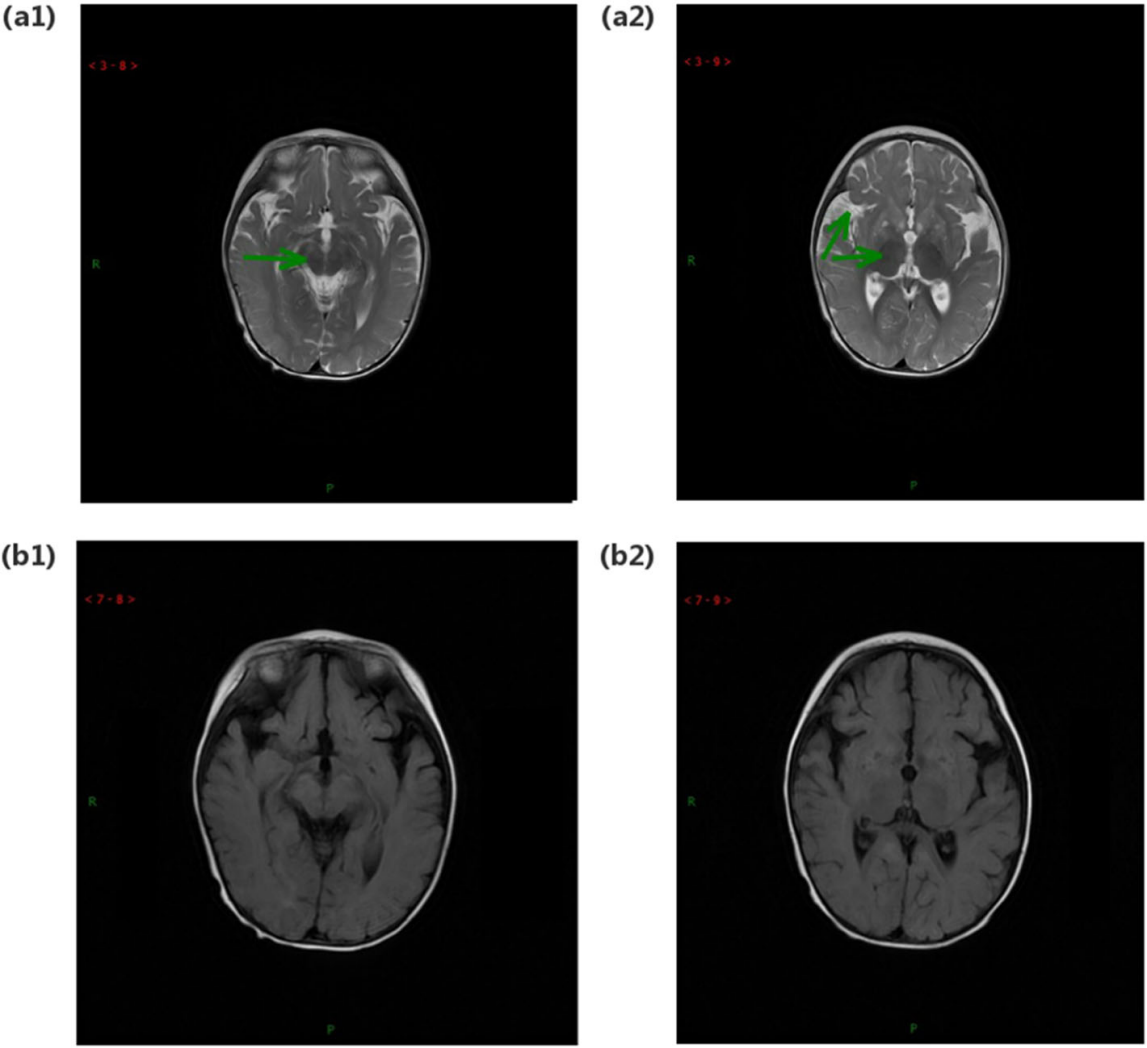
MRI and MRS of patient P4. Images (a1) and (a2) are T2-weighted images, with green arrows showing abnormal areas. (b1) and (b2) are T2-FLAIR images
